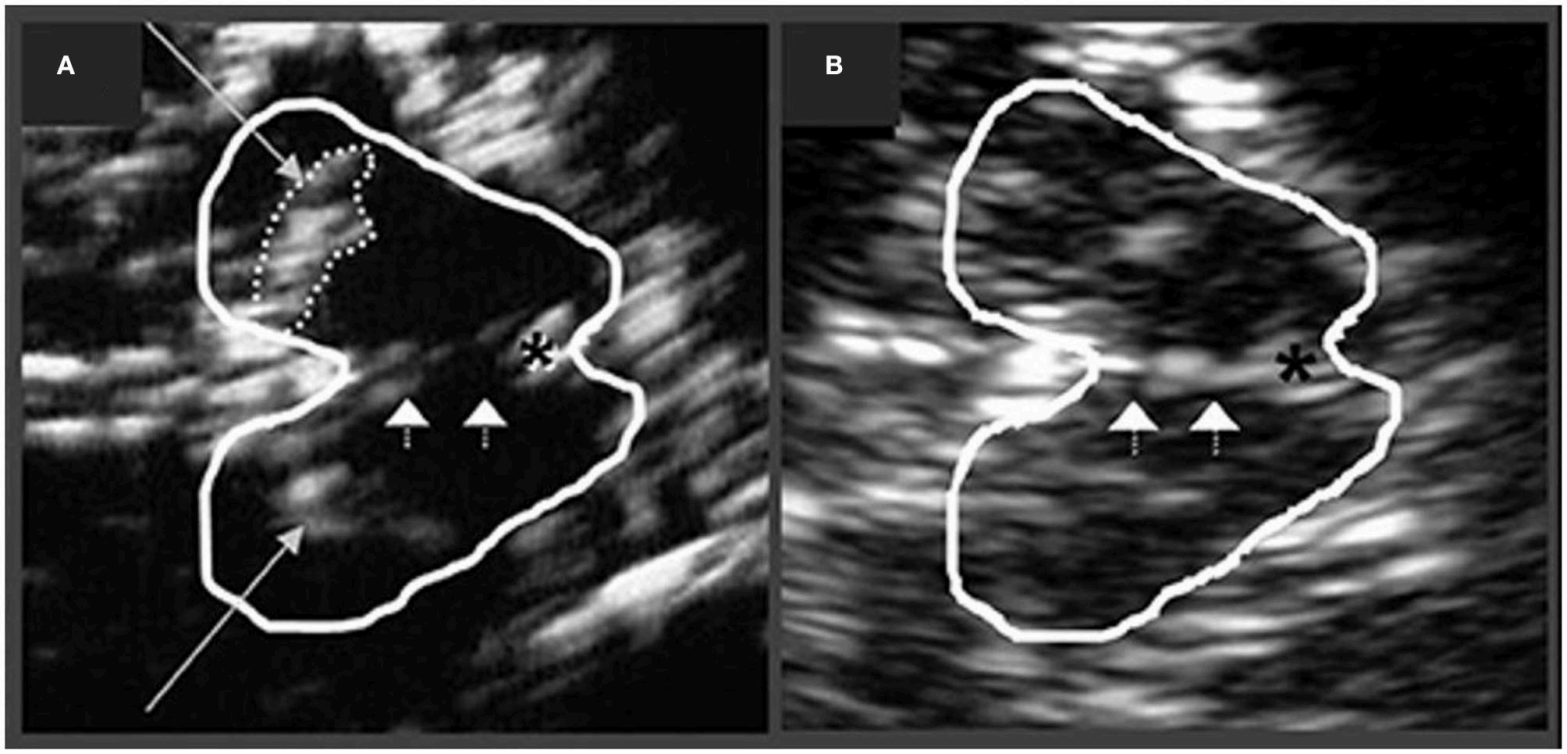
Transcranial sonographic image outlining the butterfly-shaped midbrain at the mesencephalic plane. In (A), enlarged area of echogenicity at the anatomical site of substantia nigra (long arrows) is depicted, as may be seen in Parkinson's disease patients. In addition, interrupted echogenic line of the raphe can be observed (short arrows). In (B), normal midbrain echogenicity is shown. The aqueduct is indicated by an asterisk. Figure adapted from Richter et al. (182), under the Creative Commons Attribution License (https://creativecommons.org/licenses/by/4.0/).
